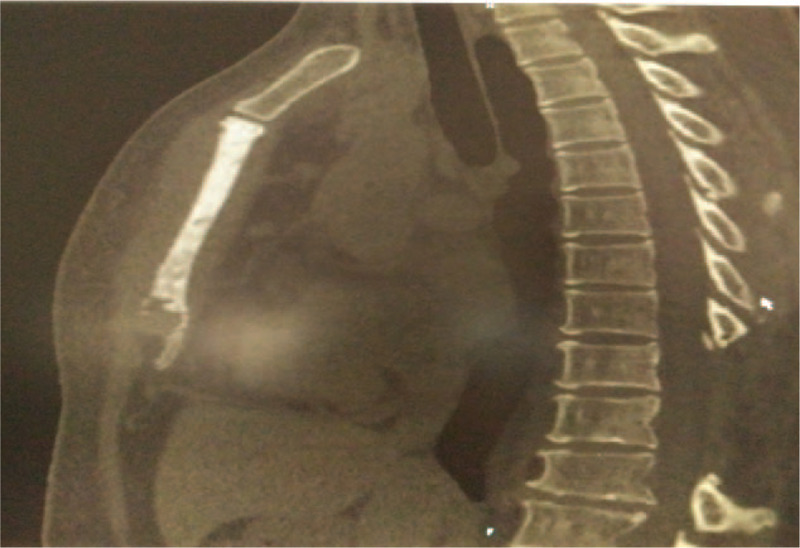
Chest CT revealed the increase of bone density in the body of sternum on February 5, 2015.
